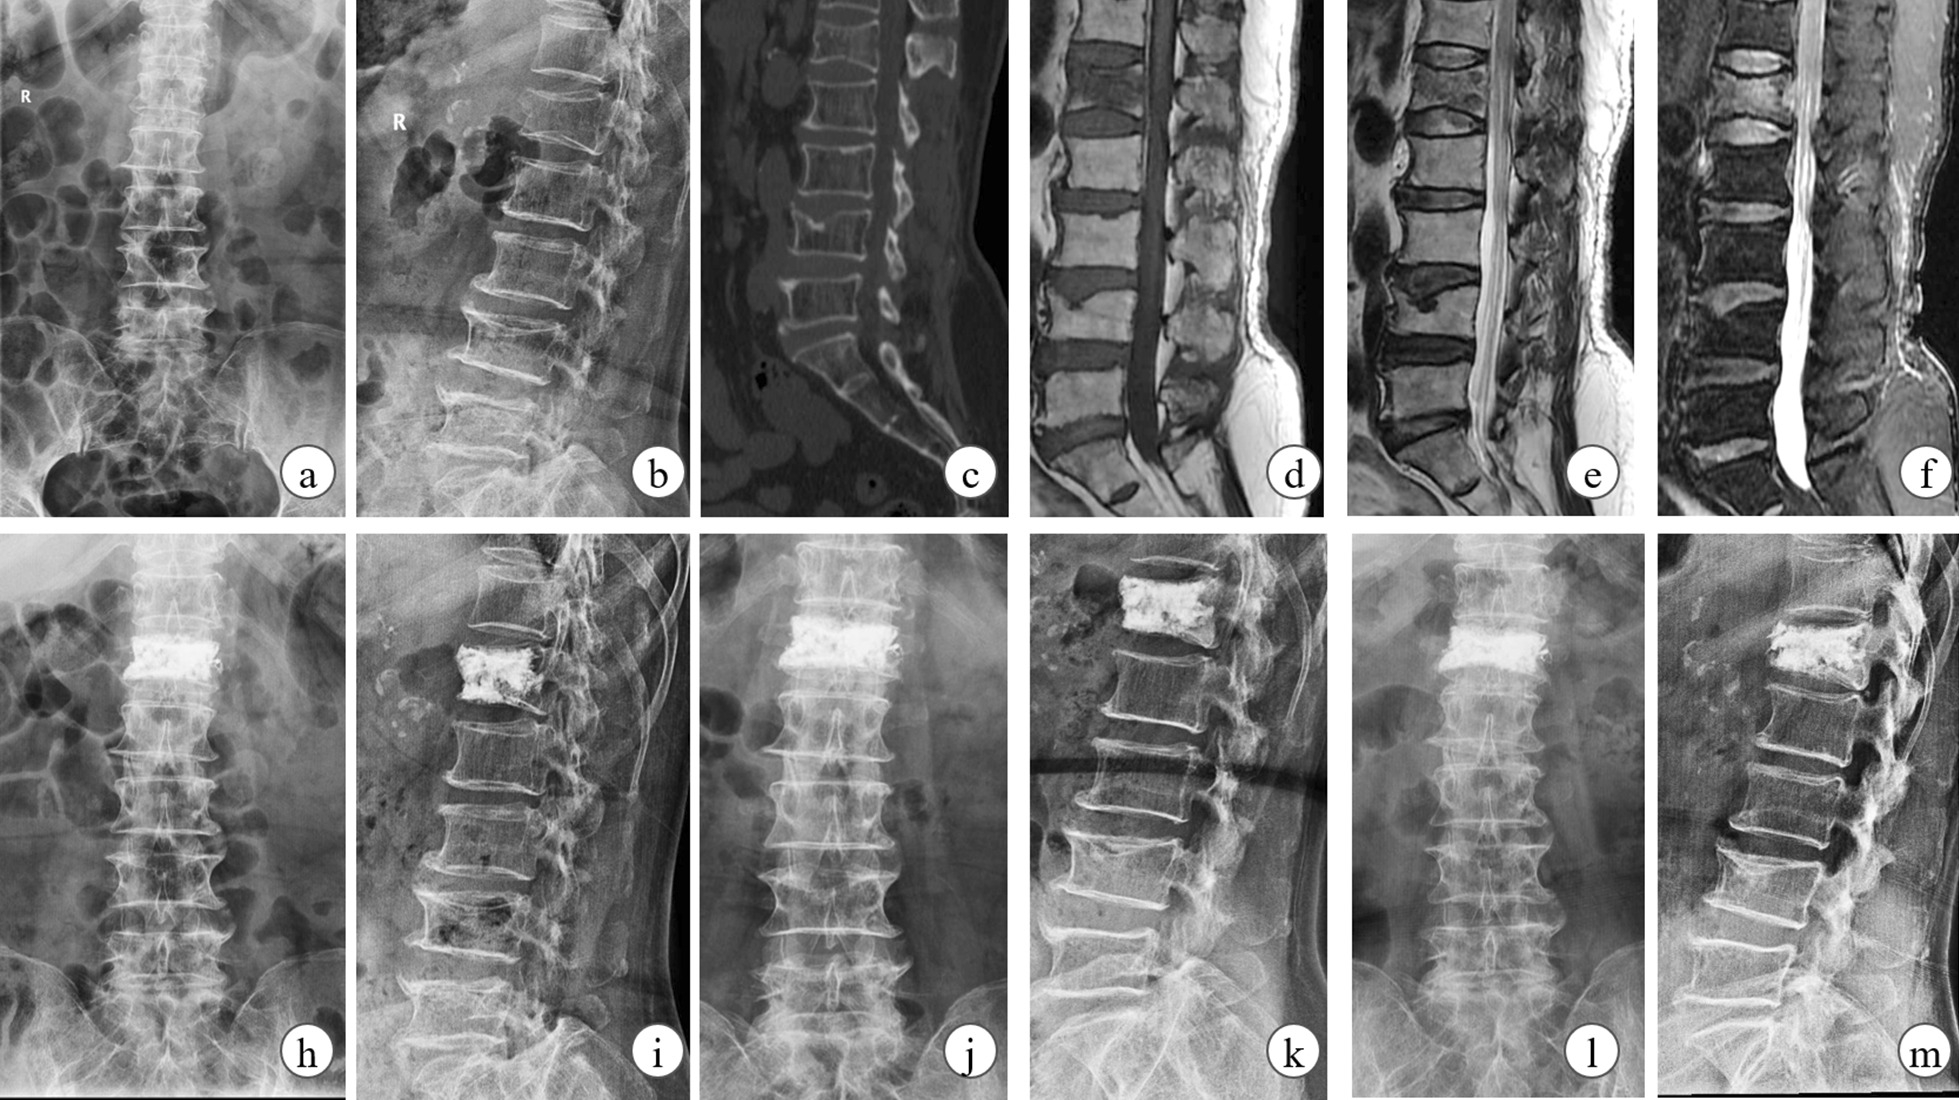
The clinical data of a 70-year-old female OVCF patient with BTP PKP surgery. The fresh fracture was located at L1 by X-Ray (a, b), CT scan (c) and MRI (df). L1 vertebra was filled up at two sides with cement and symptom was almost relieve on-month-postoperatively (h, i). Then slight collapse was observed at follow-ups on X-ray, although the cement volume and distribution were satisfied (jm)
